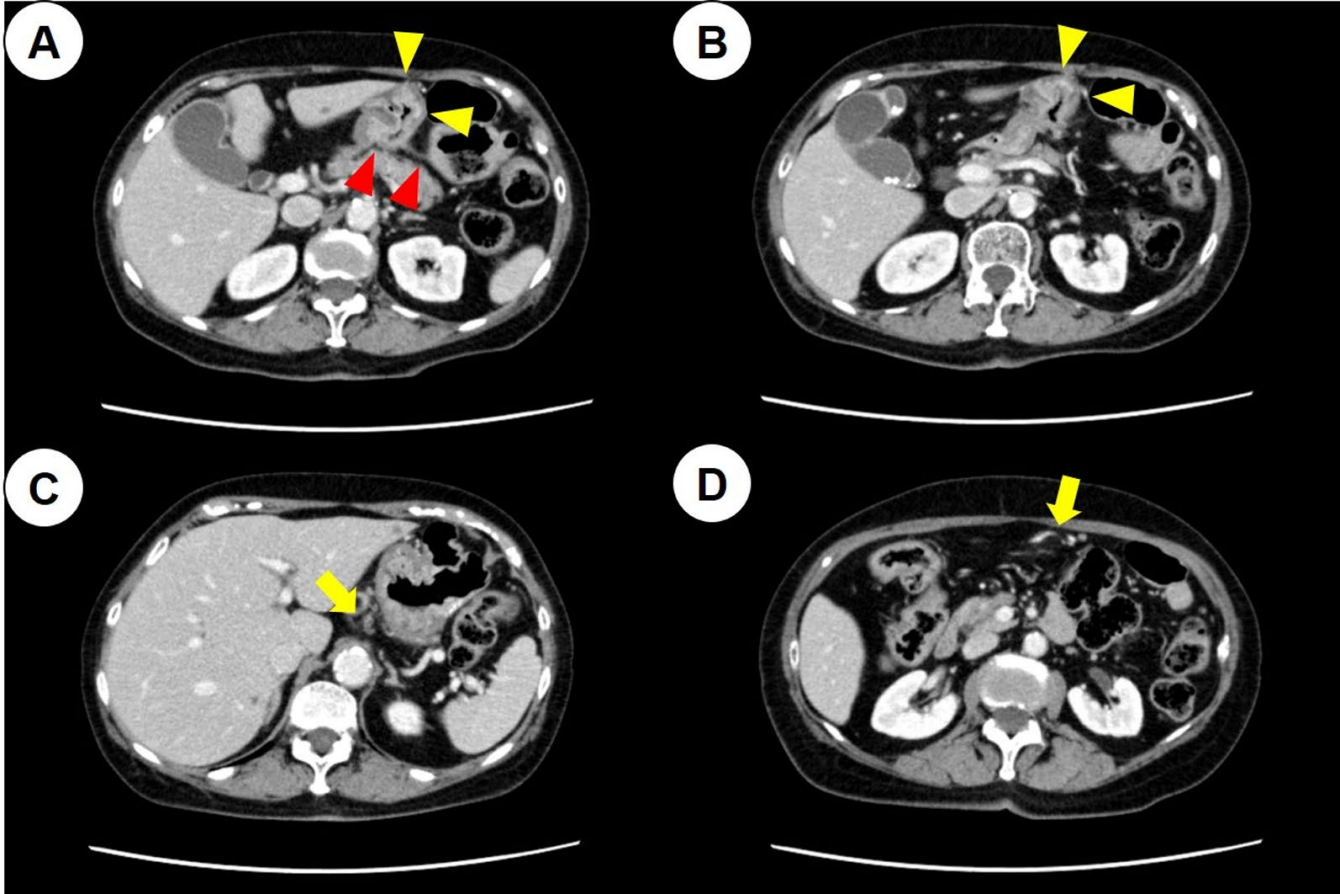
Computed tomography (CT) findings before gastrectomy. A, B CT showed that the primary lesion had regressed compared to before chemotherapy (yellow arrowheads), and the boundary between the tumor and the pancreas was clear (red arrowheads). C, D CT showed that the regional lymph nodes in lesser and greater curvatures had also shrunk (yellow arrows)
